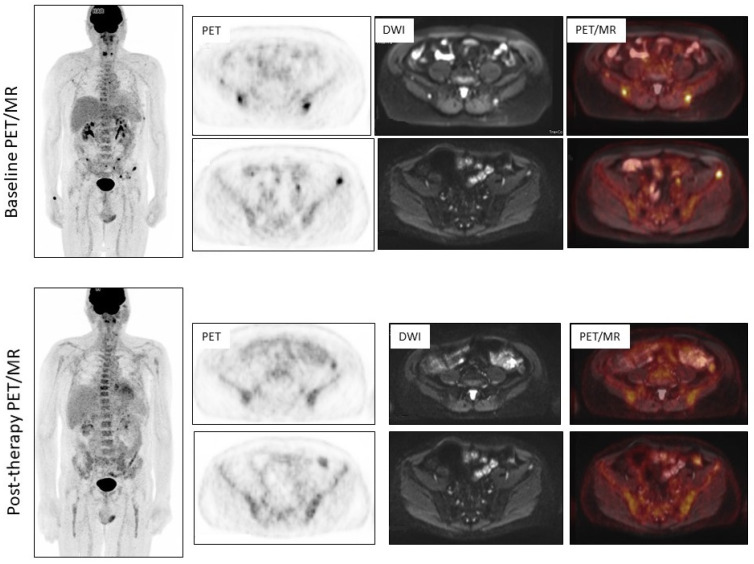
60-year-old male. Pathological fracture in C6. Bone marrow biopsy was compatible with multiple myeloma. (upper row) Baseline FDG PET/MR demonstrated the presence of multiple focal uptake in the bones, without extranodal involvement, corresponding to an increase of signal at DWI images. After induction chemotherapy (lower row), FDG PET/MR demonstrated no uptake in the site of pre-existing lesions, with the persistence of a slight signal at DWI images. Therefore, FDG–PET was conclusive for the presence of non-viable tissue.
